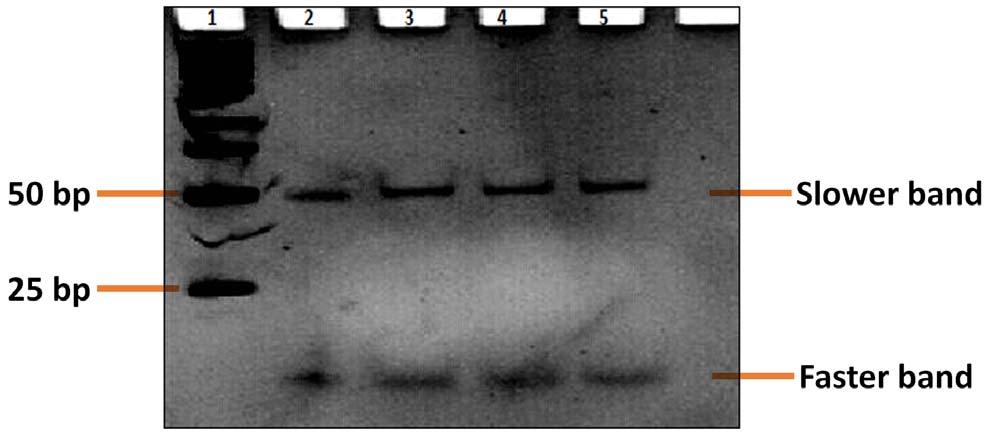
Nondenaturing 10% PAGE image of the kanamycin-binding aptamer (5′-TGG GGG TTG AGG CTA AGC CGA-3′) in the 10 mM Tris–HCl buffer (pH 7.2) containing 10 mM NaCl at 4°C (pH 7.2). (Lane 1, Marker; from Lane 2 to Lane 5: four parallel DNA samples at strand concentration of 2 μM).
